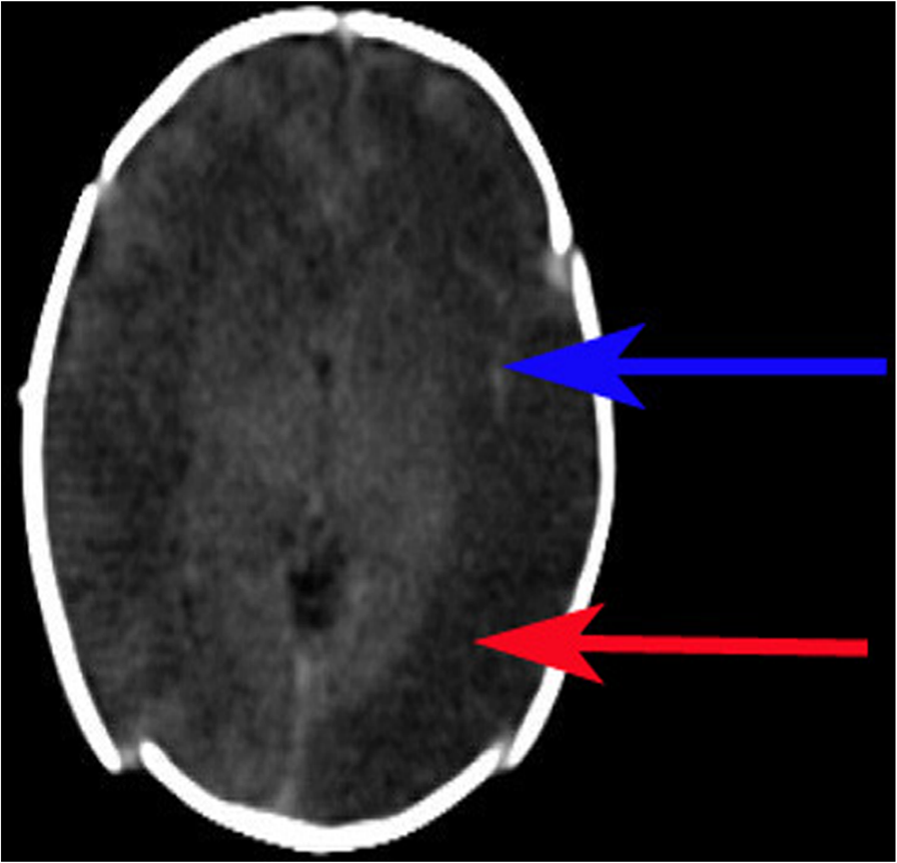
Computed tomography of head. Axial CT image show low density lesions in white matter (red *arrow*) and high density in lateral cleft pool (*blue arrow*).
